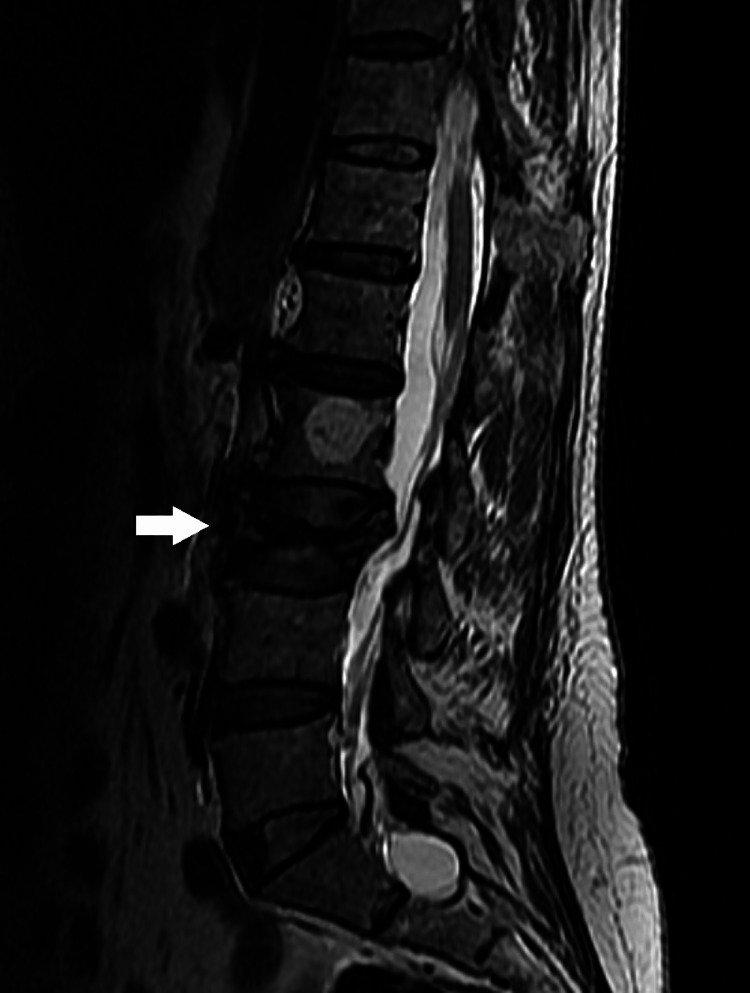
Preoperative lumbar MRI (saggital T2 view). The white arrow depicts the osteoporotic vertebral fracture (OVF) of L3.
